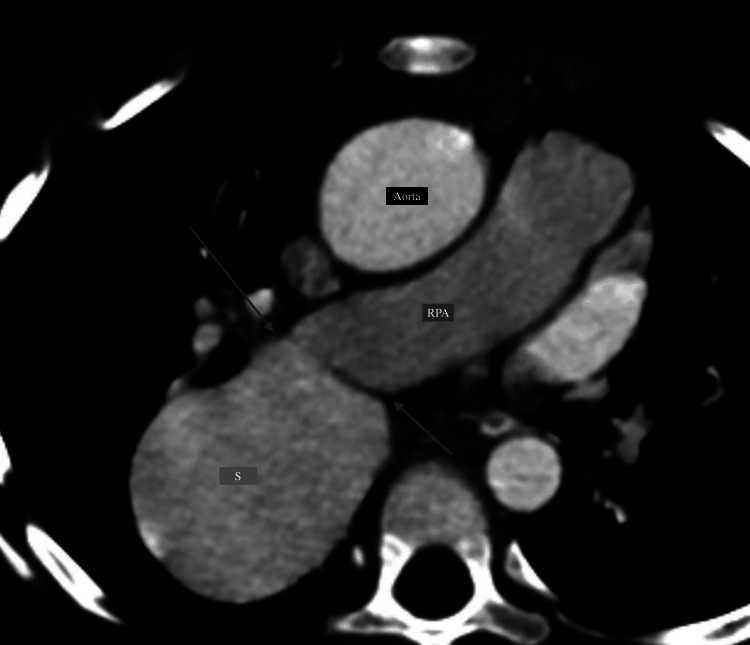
CT reformatted images (axial section) showing dilated aneurysmal sac (labeled as "S") communicating with the descending branch of the right pulmonary artery (labeled as "RPA"). The site of communication between the pulmonary artery and sac is demonstrated by the arrows. This sac is further communicating with the left atrium (shown in Figure 3).
